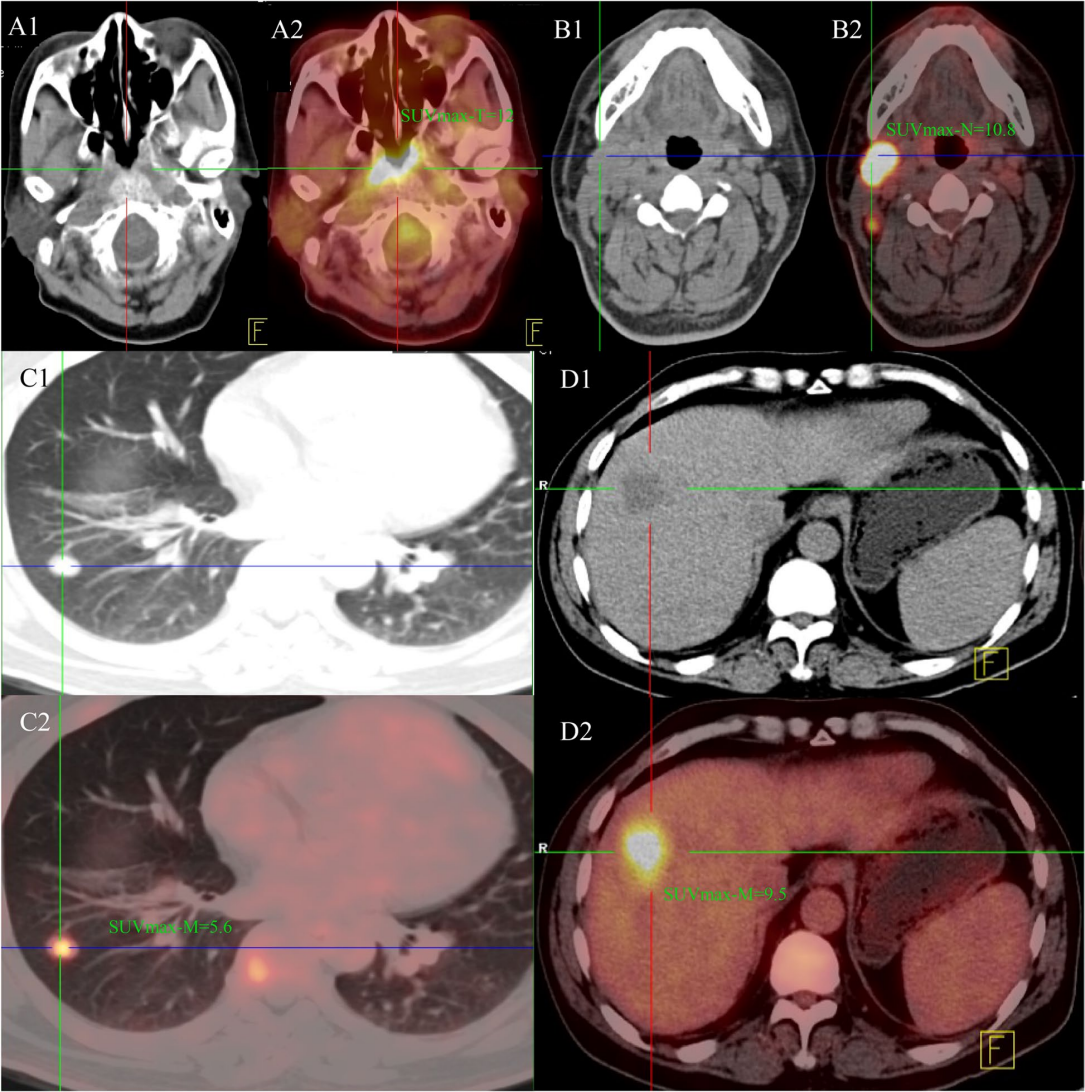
A 42 years old male with primary tumor of nasopharyngeal, right lymph node involvement, along with distant lung and liver metastasis. A1-2) SUVmax of primary tumor was 12. B1-2) SUVmax-N was 10.8, C1-2) distant metastasis of lung, SUVmax-M = 5.6, D1-2) distant liver metastasis, SUVmax = 9.5, SUVmax-M-sum = 15.1, SUVmax-M-mean = 7.6
